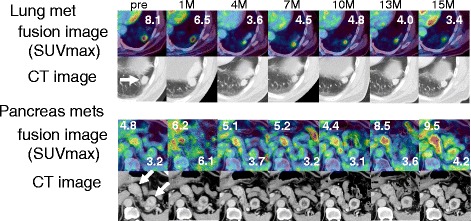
The imaging of a practice case: 70’s-year-old patient with lung and pancreas (head and tail) metastases treated by sorafenib (#1). The SUVmax of lung metastasis had suppressed during treatment, but the SUVmax of pancreas head metastasis increased before tumor was judged as PD. Fusion images were *upper lanes*. The number means SUVmax of each lesion. CT images were *lower lanes*. “Pre” refers to pretreatment evaluation; “M” refer to number of month after TKI treatment started
